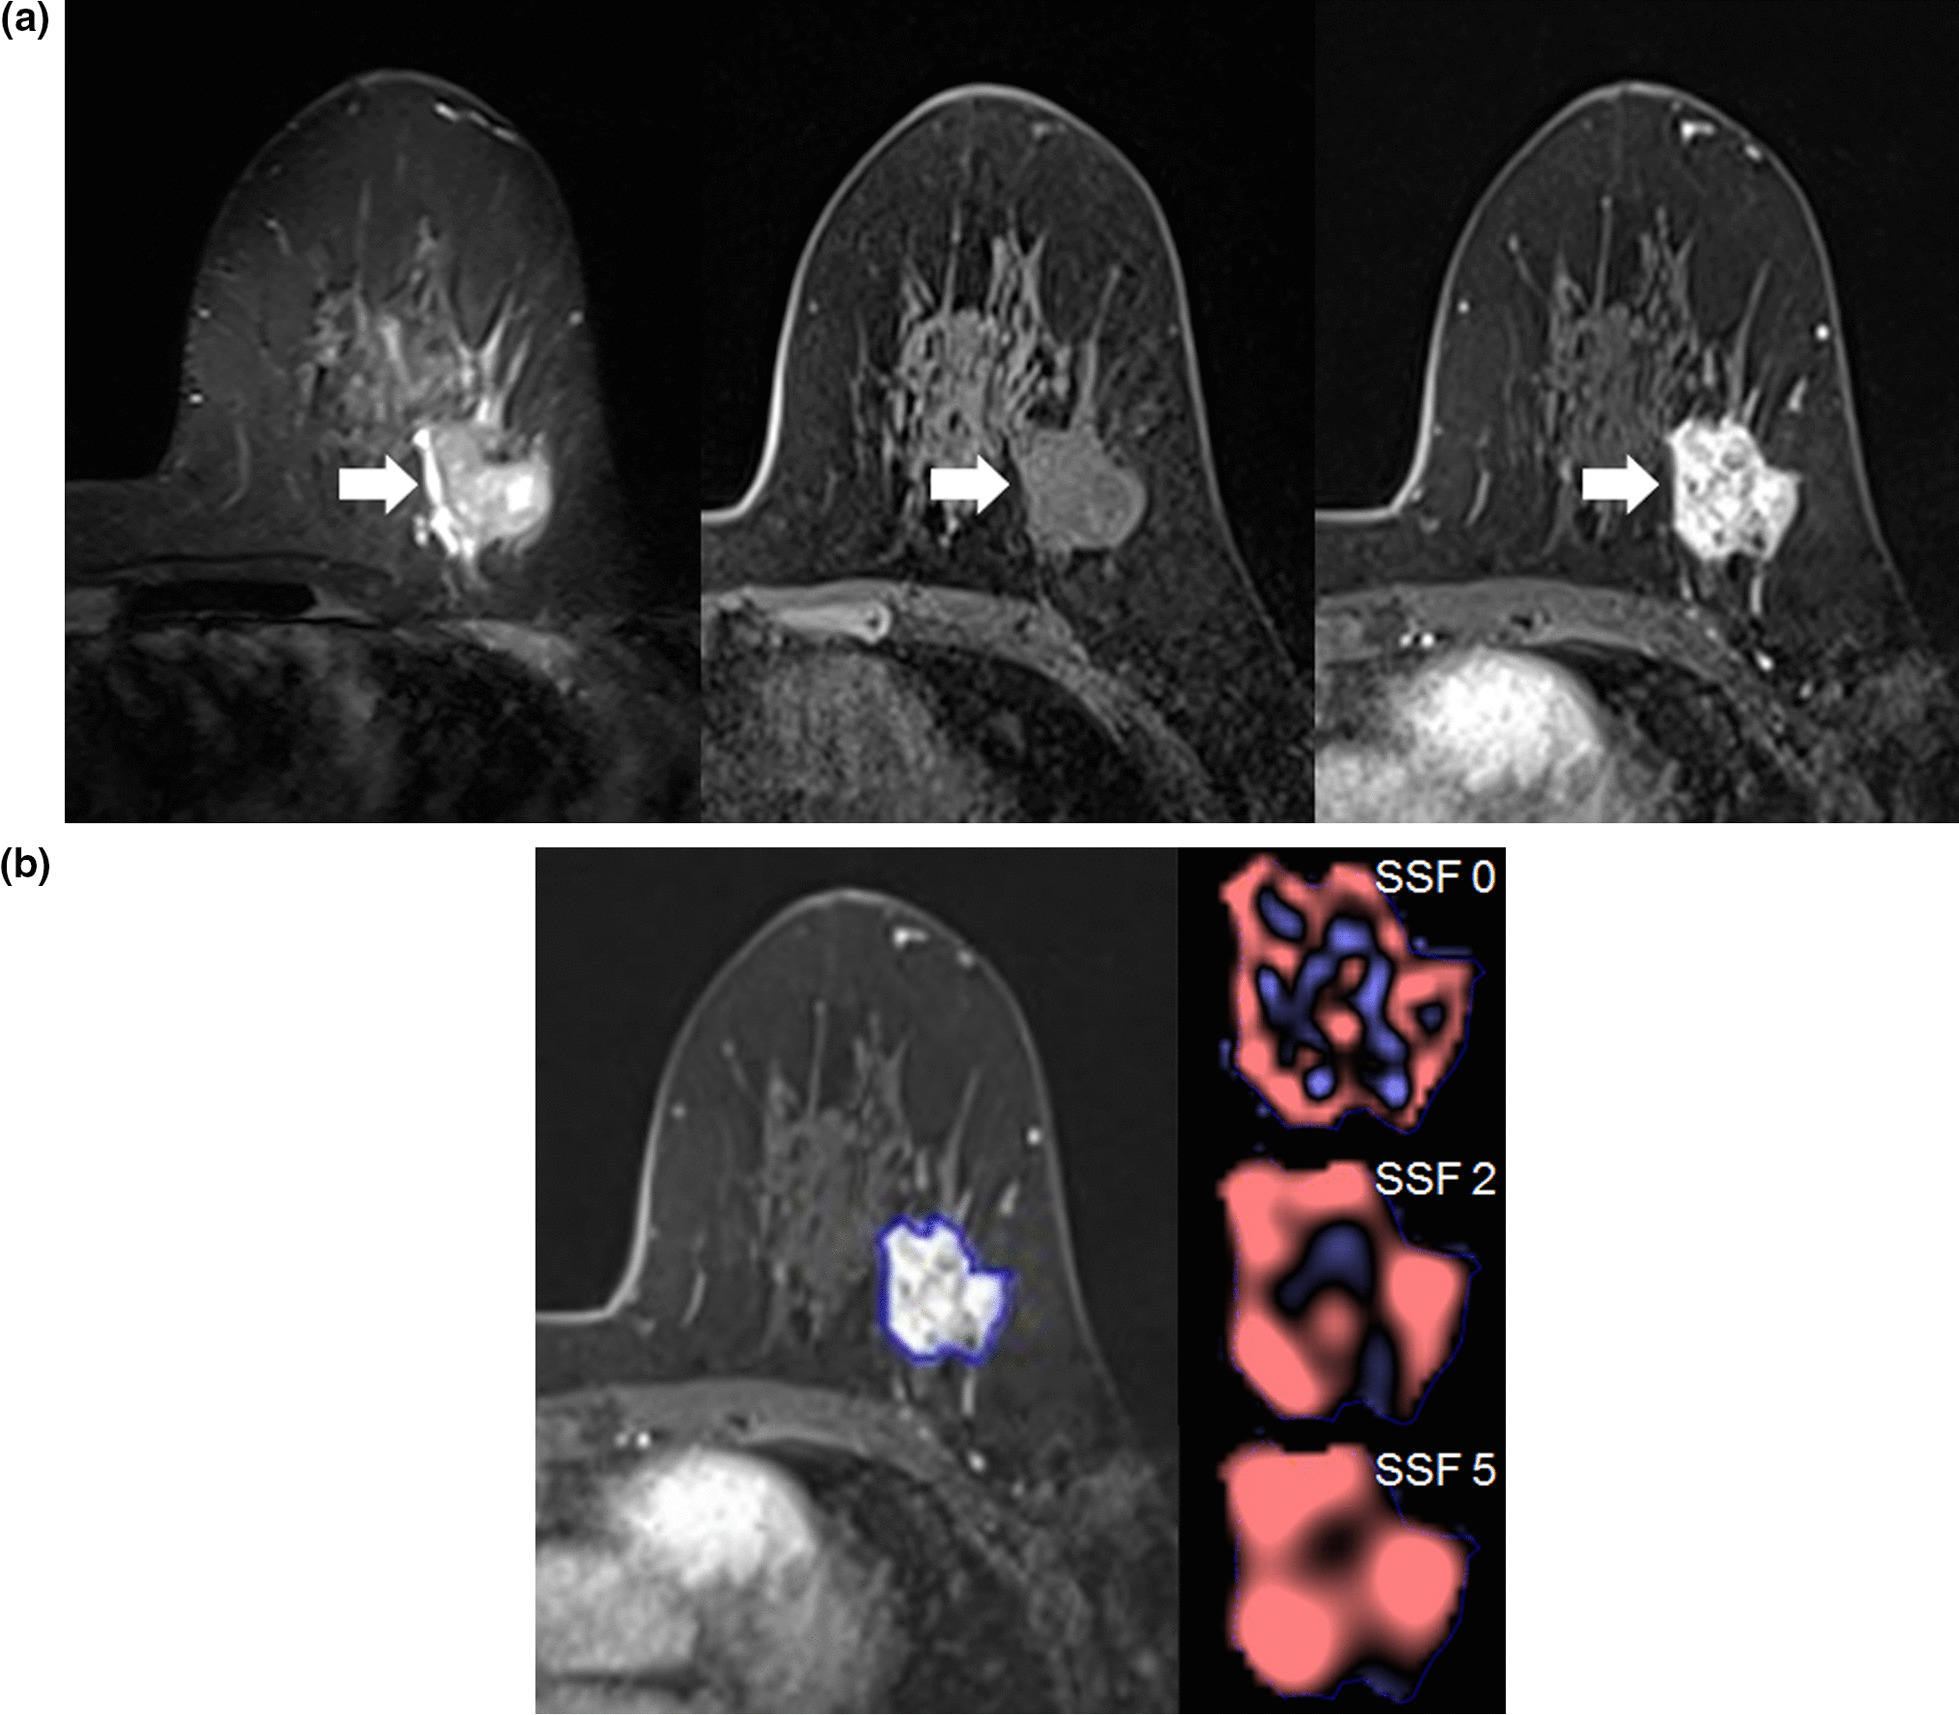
Invasive ductal carcinoma in a 61-year-old-woman. A Tumor morphology assessments were performed on T2-weighted MRI, and pre-and postcontrast T1-weighted MRI using the BI-RADS lexicon. An irregularly shaped, marginated, and heterogeneous enhancing mass (arrows) is seen. B Texture analysis was performed within a region of interest using SSFs of 0 (unfiltered texture), 2 (fine-filtered texture), and 5 (coarse-filtered texture)
